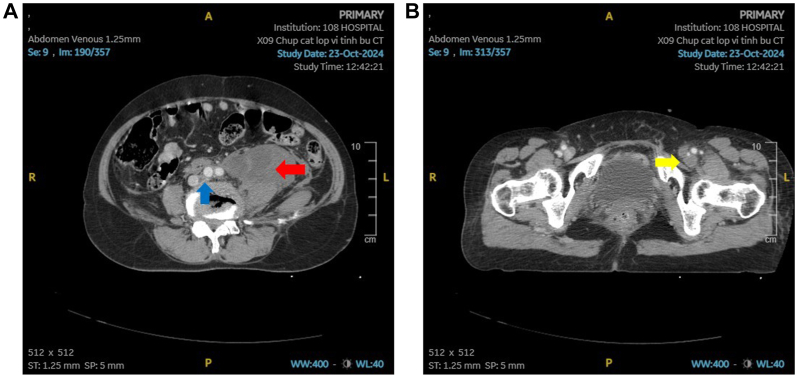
(A) Transverse computed tomography (CT) angiography of the abdomen and pelvis demonstrates a large retroperitoneal hematoma on the left (*red arrow*) compressing the left common iliac vein between the right common iliac artery and the vertebral body, resulting in complete occlusion (*blue arrow*). (B) Complete occlusion of the external iliac veins (*yellow arrow*).
